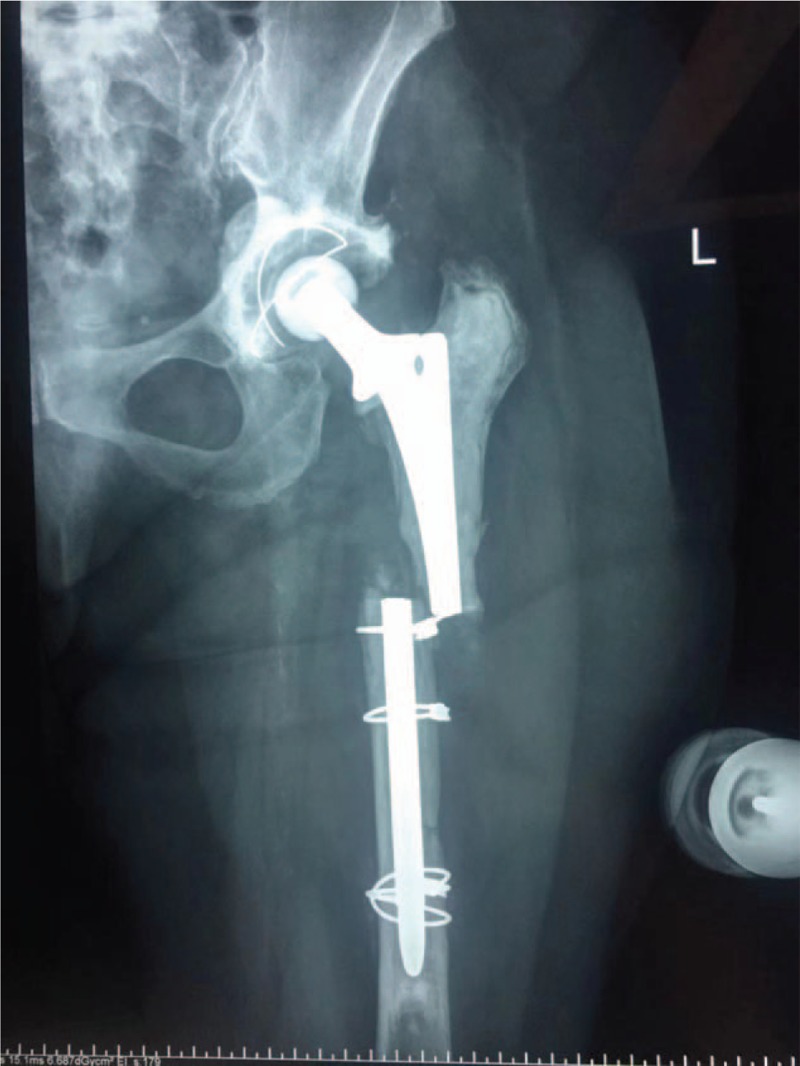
Radiograph demonstrated severe segmental bone loss of proximal femur and fracture of prosthetic stem. The femoral bone defect was evaluated carefully using the Paprosky classification system and rated as Type 3B, and the acetabular bone defect was rated as Type 2C.
